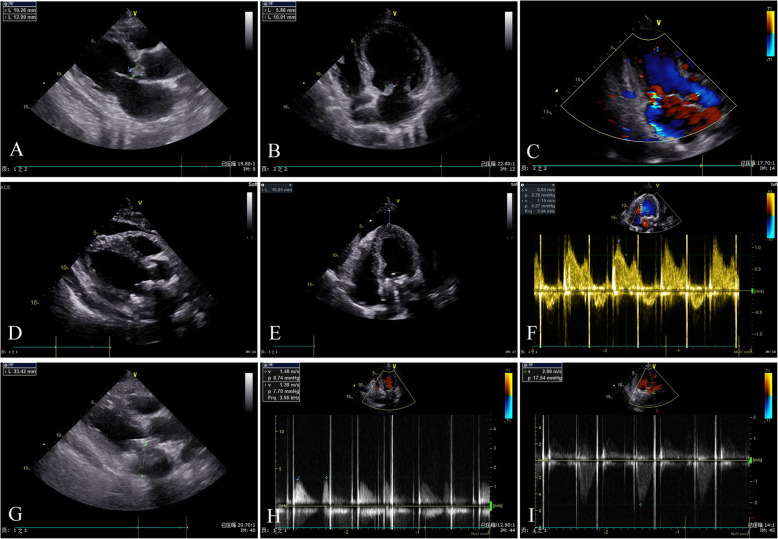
The echocardiogram findings of our patient with antineutrophil cytoplasmic antibody–positive infective endocarditis. a–c Echocardiograms reveal aortic and mitral valve vegetations (13 × 10 mm, 11 × 6 mm) and regurgitation before surgery. d–f Echocardiograms reveal normal function in prosthetic valves 14 days after aortic and mitral valve replacement and the color Doppler blood flow spectrum. g–i Echocardiograms reveal the normal function in prosthetic valves 42 days after aortic and mitral valve replacement and the color Doppler blood flow spectrum
